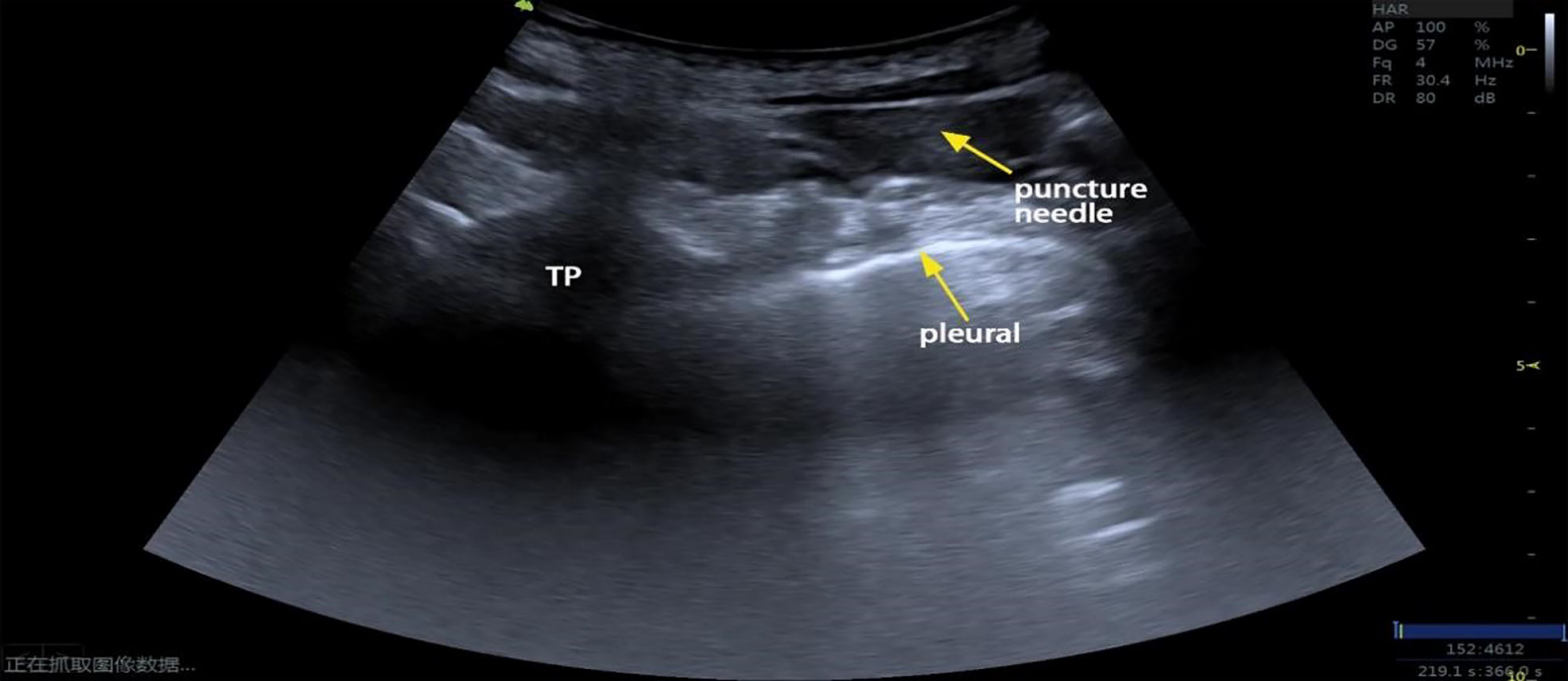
Echocardiography of ultrasound-guided TPVB (TP, transverse process).
